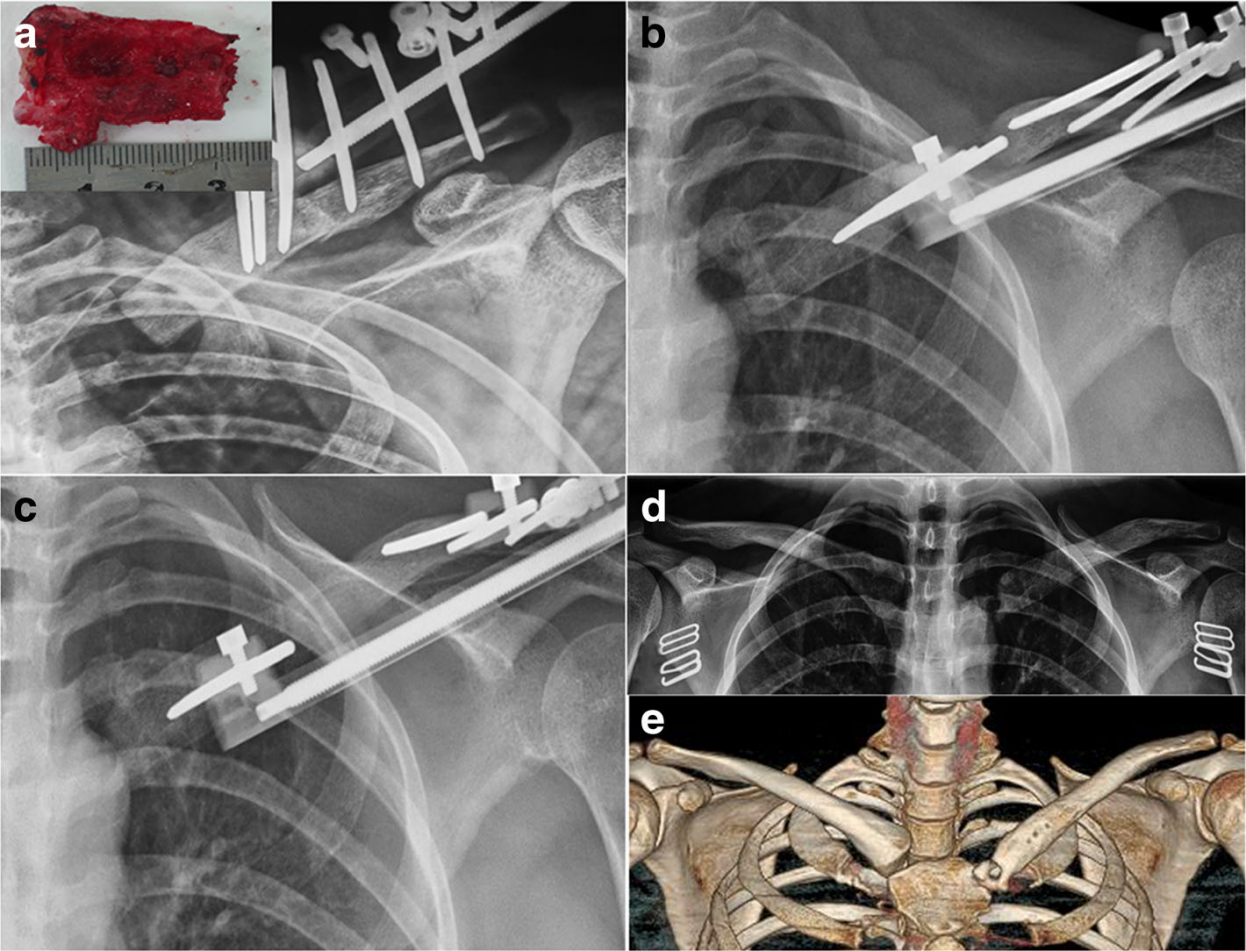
Radiographic results. a A radiograph with a 20° cephalic tilt of the right clavicle taken immediately after the surgery shows the osteotomy site and the 35-mm resection of the proximal clavicle during debridement of the SCJ. b An anteroposterior (AP) radiograph of the right clavicle taken at postoperative week 10 shows regeneration after distraction at 0.75 mm/day. c An AP radiograph of the right clavicle taken at week 26 shows consolidation of the regenerated area. d, e An AP radiograph and computed tomography scan with three-dimensional reconstruction of the right clavicle taken at week 56 after the removal of the external fixator demonstrates the sustained restoration of the clavicle length and the integrity of the sternoclavicular joint
